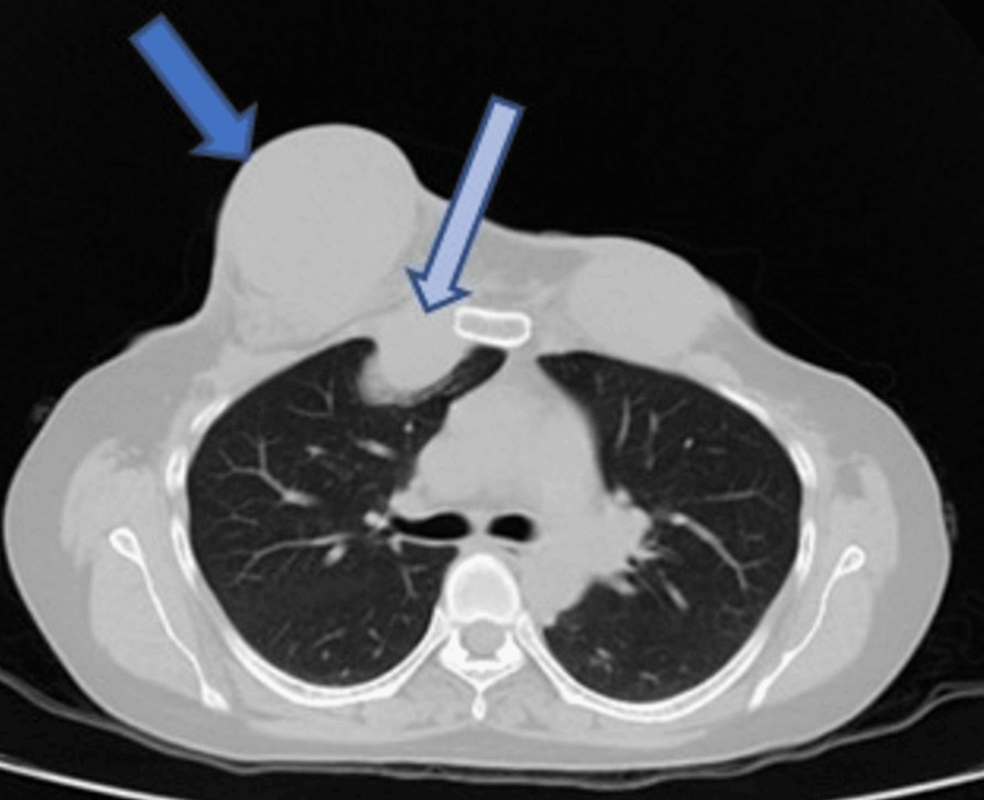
2022 CT scan. The long arrow shows tumor extension in the chest wall, while the shorter arrow indicates the tumor burden on the right side.
